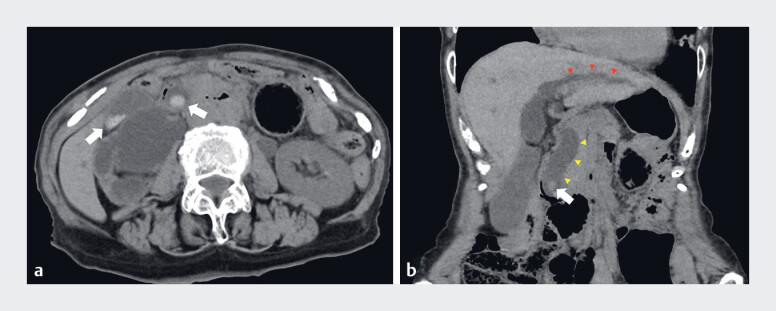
Abdominal computed tomography images showing:
